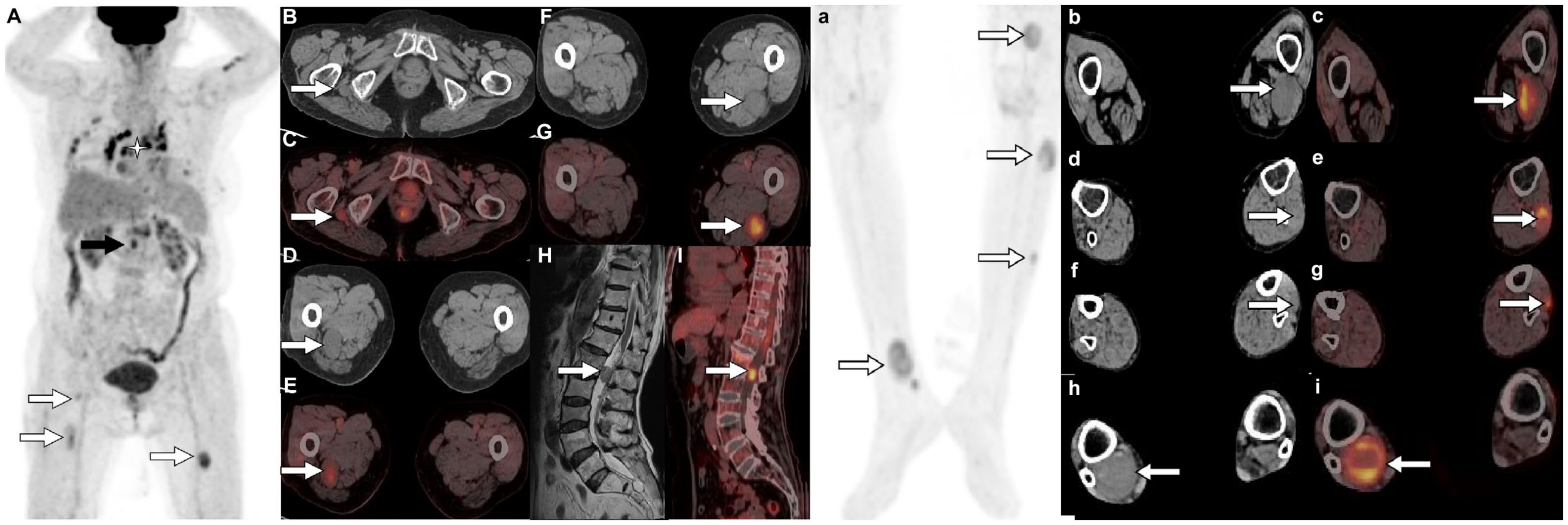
^18^F-FDG PET/CT [(A,a) Maximum intensity projection (MIP)] was subsequently performed to further evaluate the patient’s whole-body condition and revealed multiple nodules of hypermetabolic uptake in the lumbar spinal canal (black arrow) and lower limbs (white arrows). Besides, multiple high metabolic nonspecific lymph node uptakes were observed in the mediastinum (asterisk arrow). Tomographic images show multiple soft tissue density nodules with high FDG uptake on the lateral side of the right ischial tuberosity [(B) CT; (C) PET/CT fusion; arrows], upper thigh [(D) CT; (E) PET/CT fusion; arrows], middle [(F) CT; (G) PET/CT fusion; arrows] and lower left thigh [(b) CT; (c) PET/CT fusion; arrows], upper [(d) CT; (e) PET/CT fusion; arrows] and middle leg [(f) CT; (g) PET/CT fusion; arrows; SUVmax 7.5], and lower right leg [(h) CT; (i) PET/CT fusion; arrows]. In addition, isomuscular signal on T1WI (H, arrow) and high metabolic uptake nodule on PET/CT (I, arrow) were also observed in the posterior spinal canal of the first lumbar spine.
